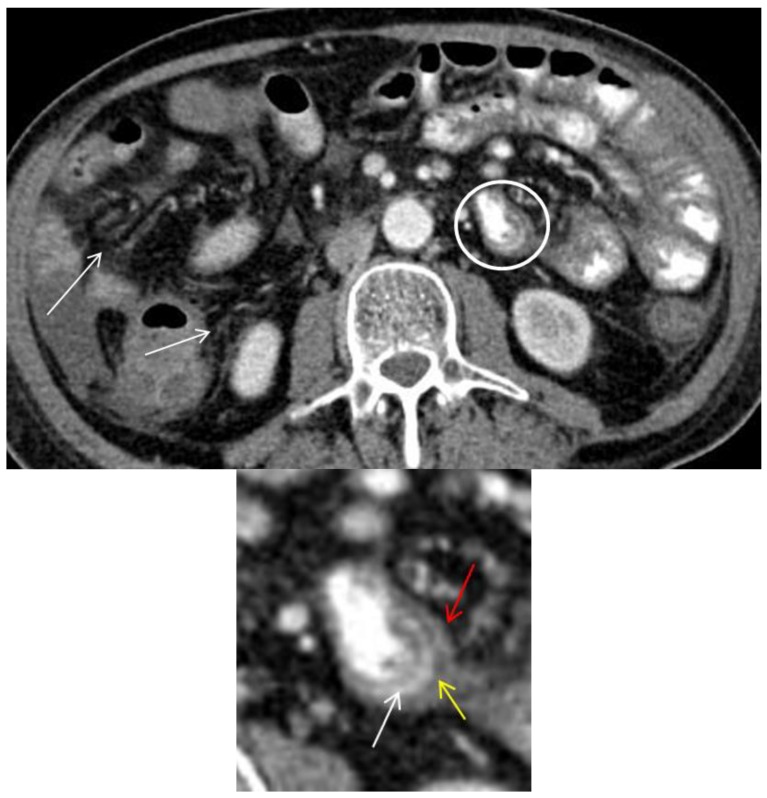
Axial CT image shows circumferential small bowel wall thickening (>3 mm) with trilaminar mural stratification in the small-bowel (circle and magnified image) with serosal enhancement (red arrow), low intramural attenuation (yellow arrow) and mucosal enhancement (white arrow) [2,13]. Low intramural attenuation can represent edema, inflammatory infiltrate or fat [13]. Stranding of the mesenteric fat is often observed in GIT-GVHD [2,6] (arrows). Mural stratification is a non-specific sign of bowel inflammation and has been shown to correlate with clinically active disease as opposed of a more homogenously enhancement due to fibrosis [14,15]. In this patient, three different patterns of mural stratification and mural enhancement were present in separate segments (Figure 2 and Figure 3).
